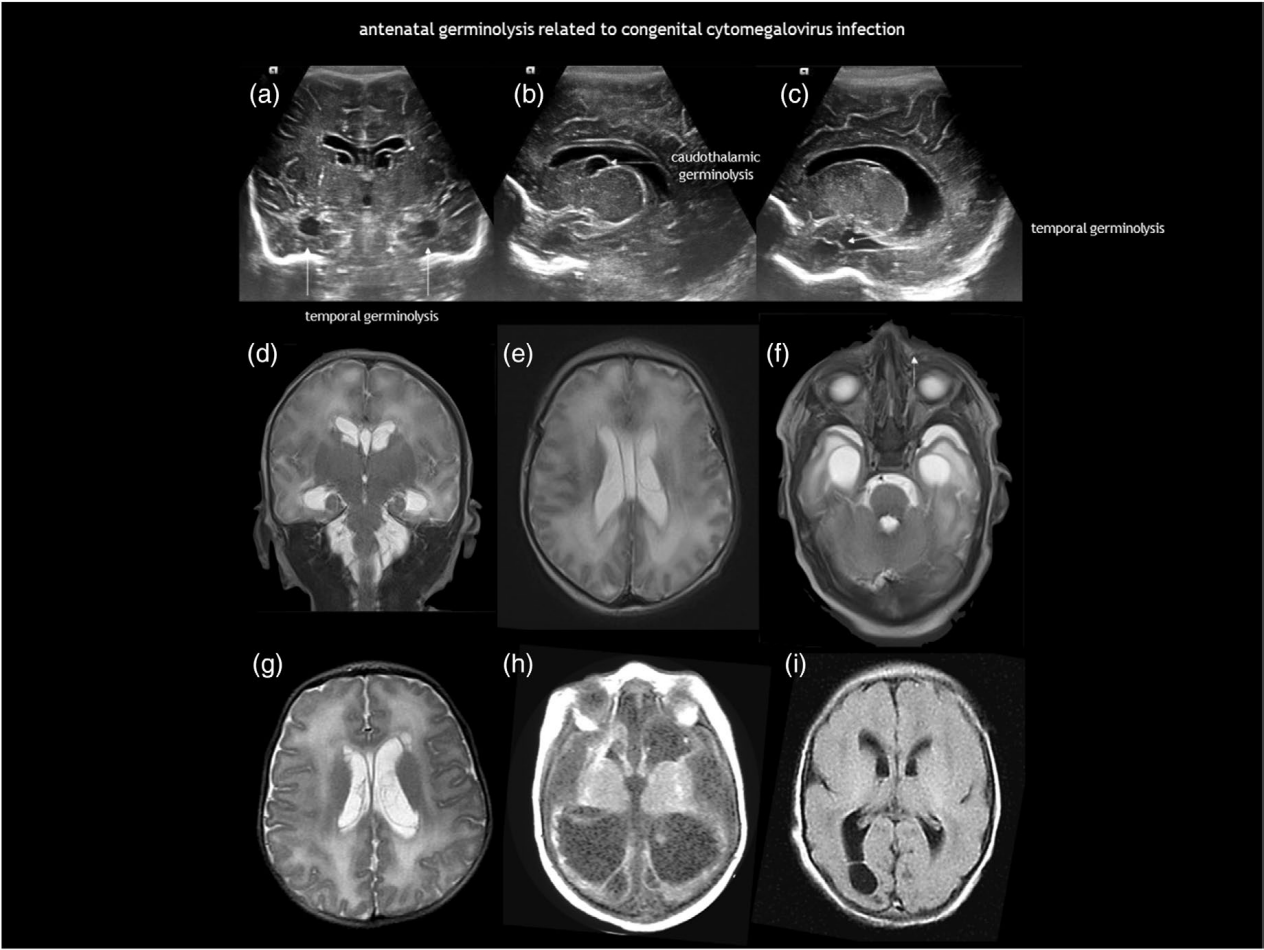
Antenatal germinolysis related to congenital cytomegalovirus (CMV) infection. (a–c) Cranial ultrasonography (CUS) images of an infant with confirmed congenital CMV. (a) Coronal. (b,c) Parasagittal. (d–f) Brain magnetic resonance imaging (MRI) of an infant with confirmed congenital CMV. (d) Coronal T2. (e,f) Axial T2. CUS more clearly reveals caudothalamic germinolysis; however, temporal horn involvement is subtle on CUS and better depicted on MRI. (g) Axial T2 MRI of another infant with congenital CMV showing parafrontal and caudothalamic germinolysis. (h) Coronal T1 MRI of a third infant with congenital CMV, demonstrating severe ventriculomegaly, cortical thinning, smooth cortex, periventricular and central grey nuclei calcifications, and occipital horn septation. (i) Axial T2 fluid‐attenuated inversion recovery MRI of a fourth neonate with congenital CMV, showing septation and adhesion in the right occipital horn.
